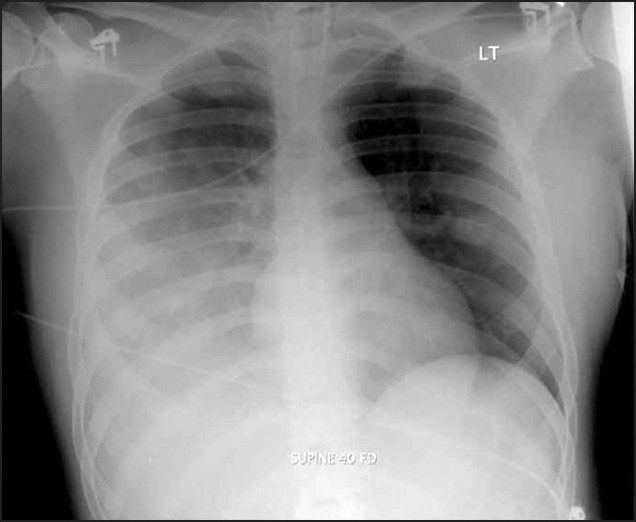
Chest x-ray on admission to ICU, showing right consolidation and pleural effusion.
